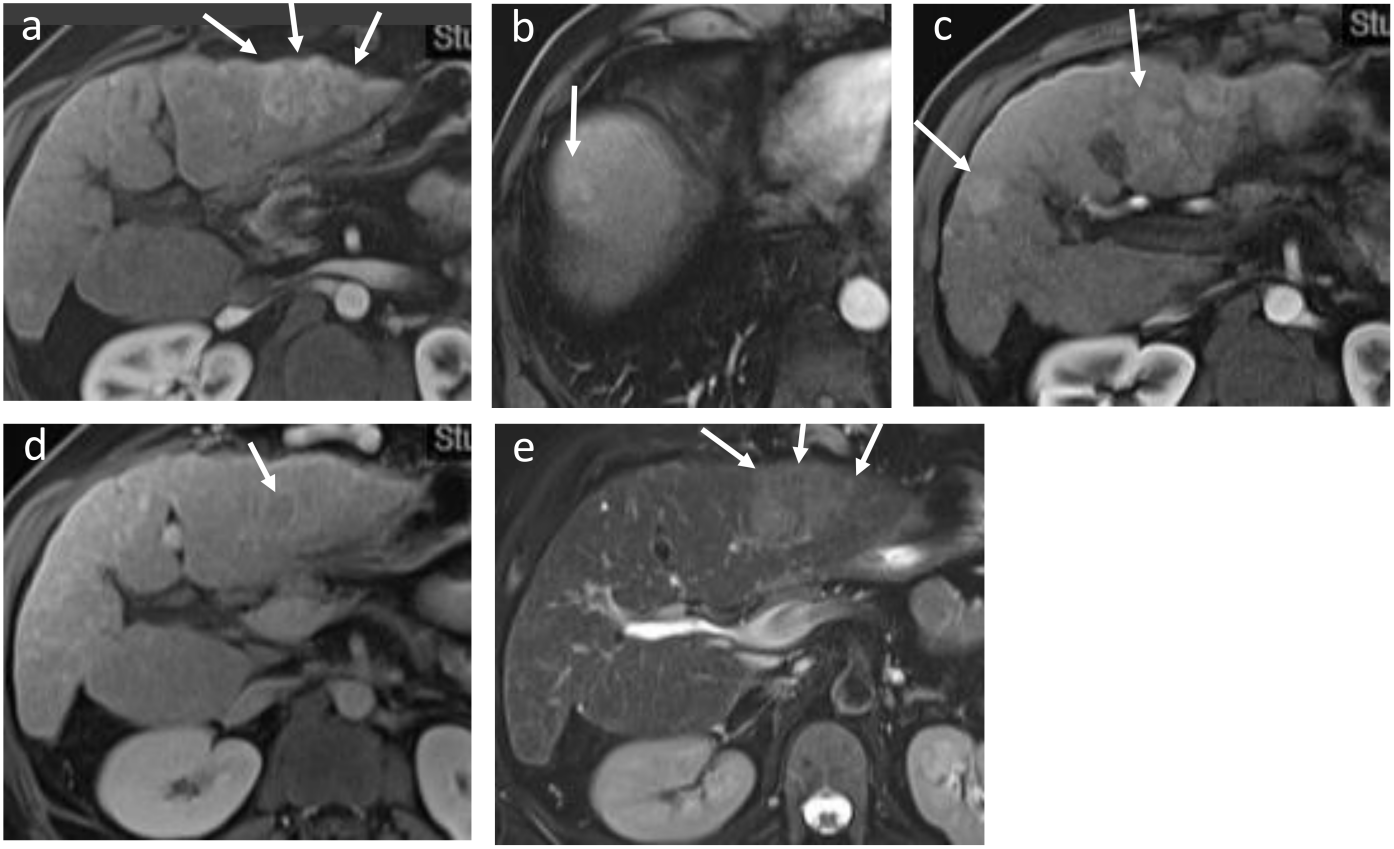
Initial MRI showing multiple hypervascular lesions and no macrovascular tumoral invasion: a main lesion (6 cm in diameter) with late washout on portal phase and slightly hyperintense T2 in the left lobe, and multiple smaller lesions in the right lobe. (A) Sequence T1 with injection (arterial phase): main lesion in the left lobe; (B) Sequence T1 with injection (arterial phase): multiple hypervascular lesions in the hepatic dome and (C) in the segment IV and V. (D) Sequence T1 with injection (portal phase): late washout of the main lesion. (E) T2 sequence: main left lesion slightly hyperintense T2.
